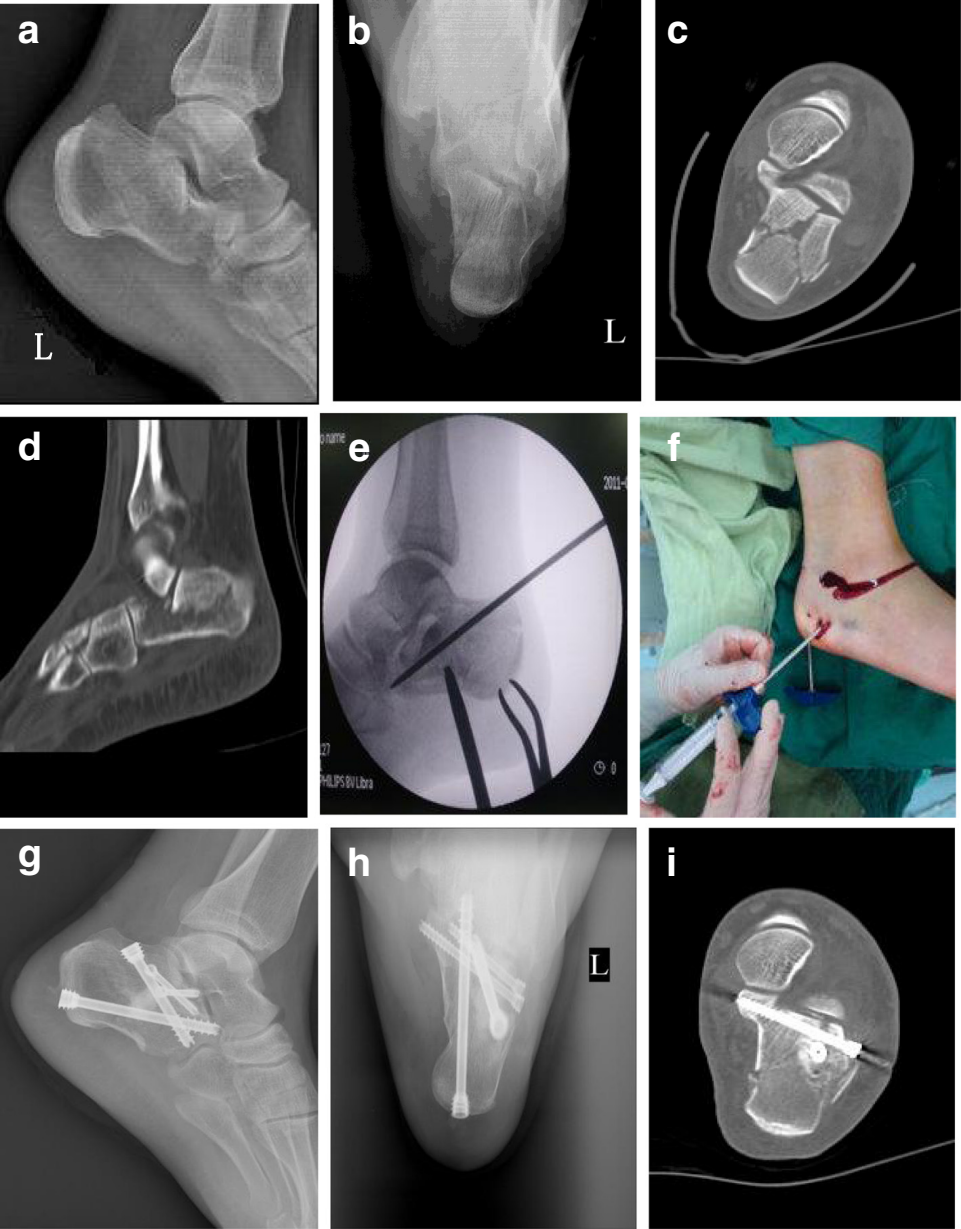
A 42-year-old female patient suffered left lateral displaced intra-articular calcaneal fracture caused by a fall from a height. Preoperative X-ray: lateral view (a) and axial view (b) showed significantly decreased calcaneal height/length, Bohler angle, Gissanes angle, and significantly increased calcaneal width. A preoperative CT-scan (c, d) showed a Sanders Type-II. A calcaneal fracture with collapse of the subtalar articular surface. Intraoperative fluoroscopy (e) showed the reduction of the subtalar joint surface through two Schanz pins that were used to lever and percutaneously push up the depression of the subtalar joint surface. The CSC was injected into the void of the body (f). Postoperative X-ray: lateral view (g) and axial view (H) showed calcaneal anatomical reduction. Postoperative CT-scan (i) showed the reduction of the articular surface was satisfactory, however, the restoration of calcaneal width was still dissatisfactory
